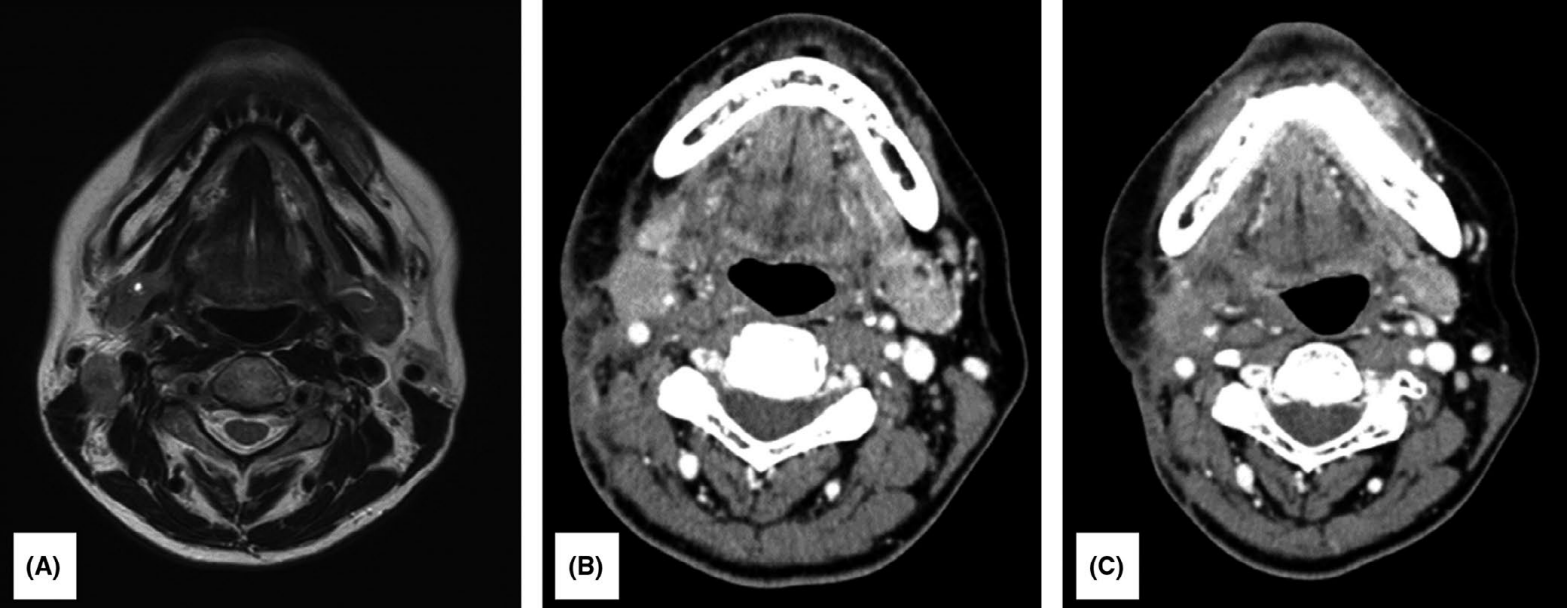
(A) Magnetic resonance image taken 7 months after tongue cancer surgery. The right superior‐internal jugular vein lymph node had become swollen with a minor axis of approximately 12 mm, and the T2‐weighted image shows a region with moderate signal intensity that included a part of the low‐signal region. (B) Computed tomography image taken 3 months after right neck dissection. The right submandibular lymph node had become swollen with a minor axis of approximately 15 mm, and the boundary with the surrounding area was unclear. (C) Computed tomography image taken after chemoradiotherapy. No change in the size of the right submandibular lymph node was observed, and the boundary with the surrounding area was unclear
